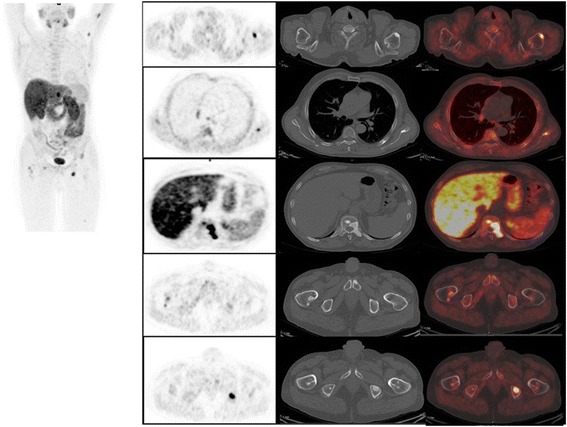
[^18^F]FMCH PET/CT and bone metastasis in a patient with wide spread disease
